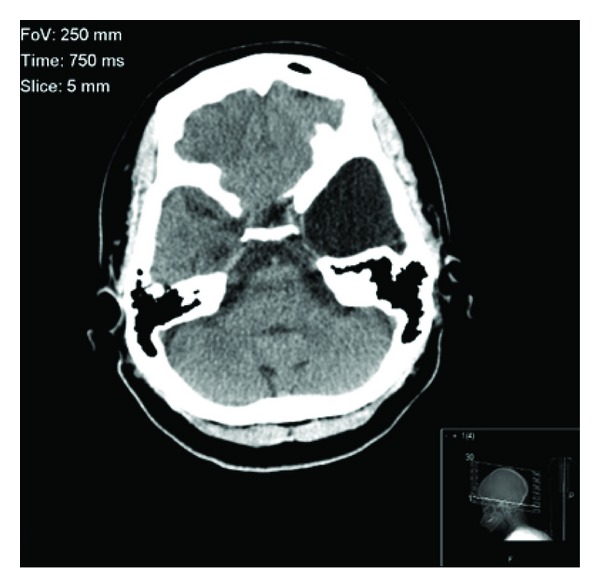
Cranial CT without contrast in the horizontal plane, showing an arachnoid cyst in the region of the left temporal lobe.
